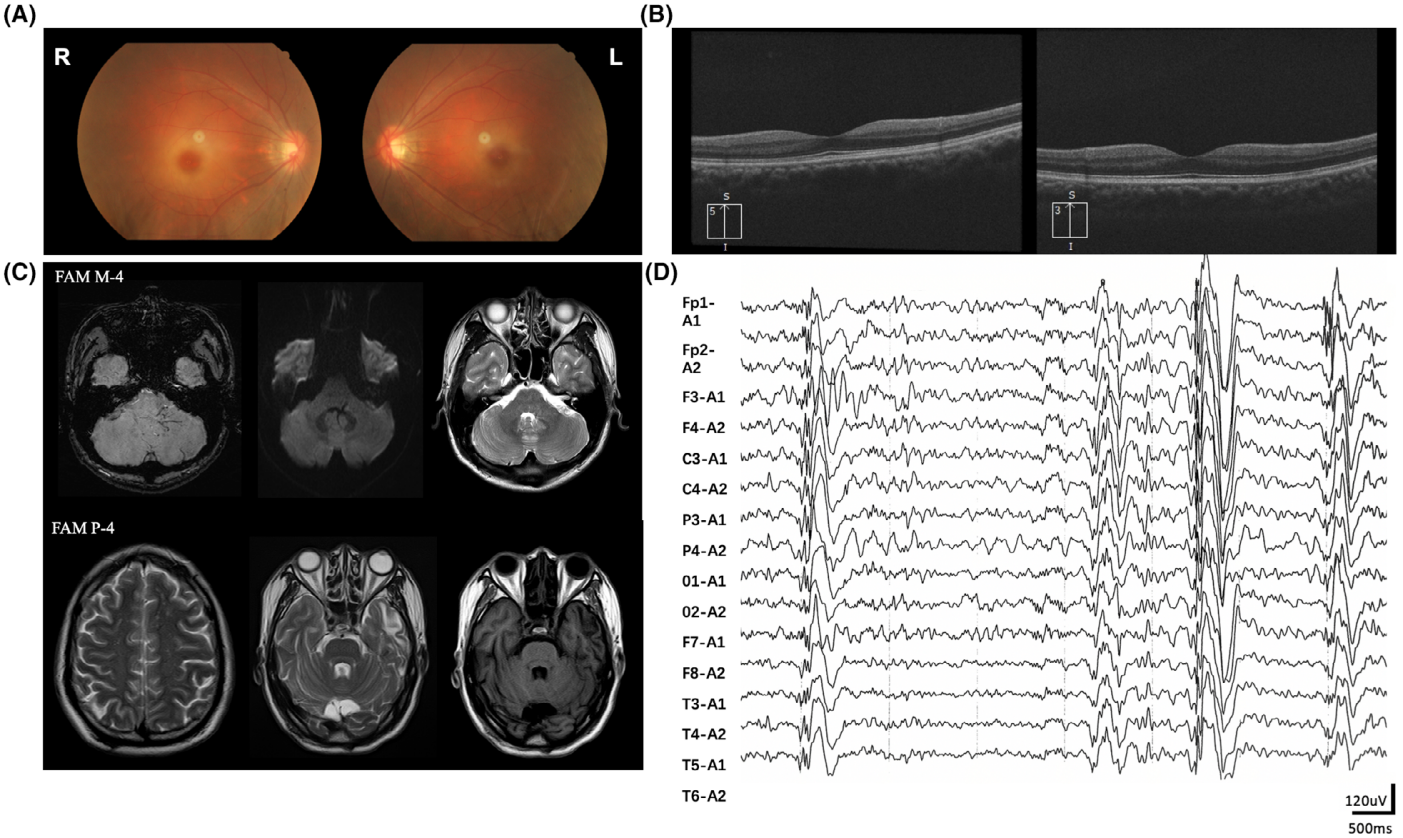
Auxiliary examination results of patients. (A) Color fundus photographs of the patient Fam F‐3 showed bilateral cherry‐red spots in the macular. (B) Optical coherence tomography of patient Fam B‐6 and Fam L‐3 showed thinning of the retinal nerve fiber layer. (C) Brain MRI of the patient Fam M‐4 exhibited several linear dilated veins in the left cerebellar hemisphere and pontic arm. The diffuse weighted imaging (DWI) sequence revealed flow shadows. On T2‐weighted image (T2WI), the lesion was illegible. Brain MRI of Patient Fam P‐4 showed several spot‐like T2WI hyperintensities in the bilateral frontal and parietal lobes, as well as patchy T2WI hyperintensities and T1‐weighted image hypointensities in the left temporal lobe. (D) EEG of the patient Fam P‐4 showed generalized polyspike and polyspike‐slow waves.
